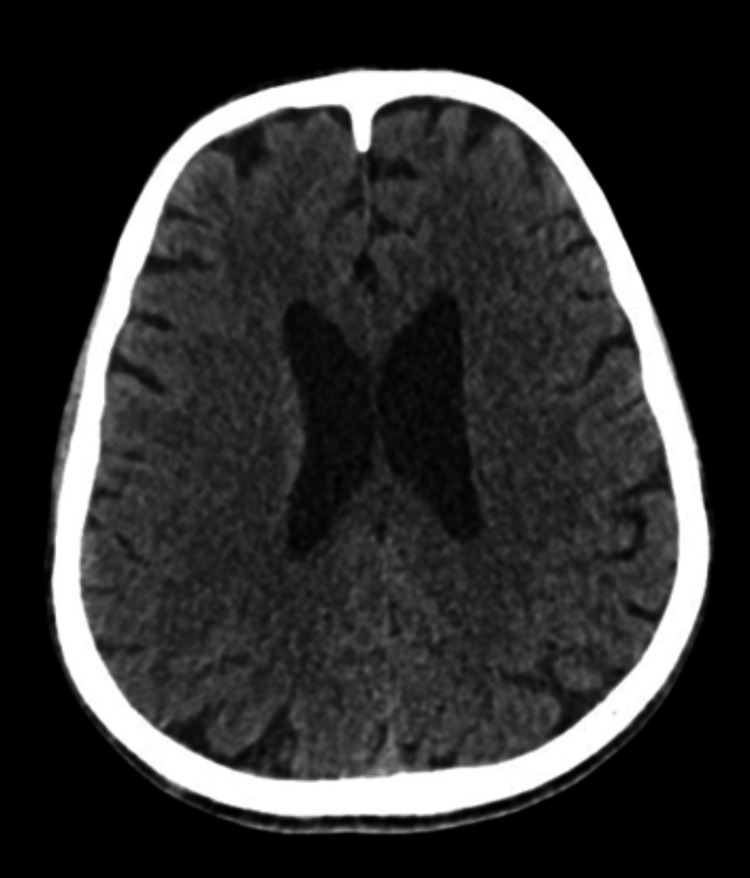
Noncontrast CT of the head (sagittal view) showing no acute abnormalities
